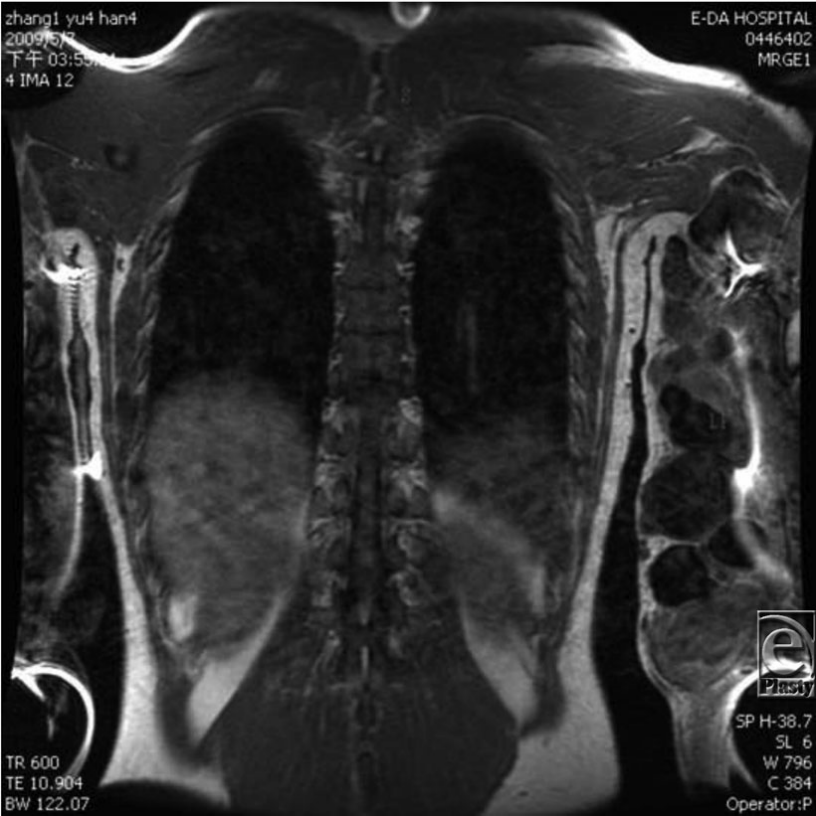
Magnetic resonance imaging revealed an extensive, diffuse arteriovenous malformation involving all compartments of the upper limb. There was no skeletal hypertrophy.
